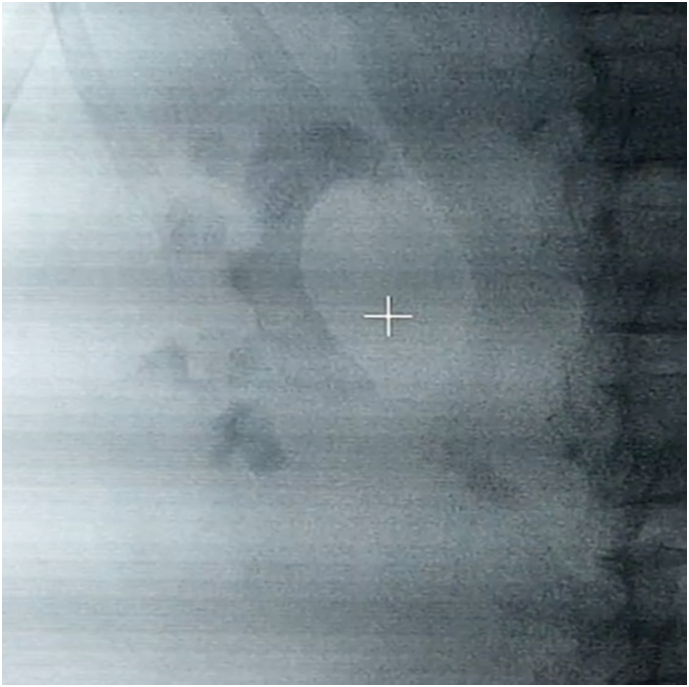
Non-contrast x-ray showed an almost complete staghorn stone in the right kidney and a sizeable upper ureter stone.
